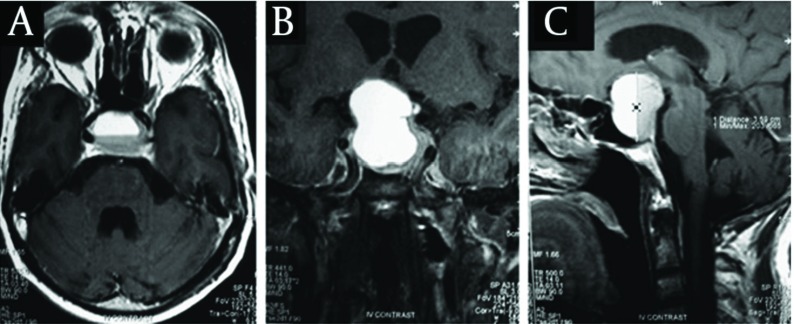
Preoperative Axial (A), Coronal (B), Sagittal (C) Magnetic Resonance Imaging Scan Following Contrast Injection of Patient Documenting a Suprasellar Pituitary Adenoma
